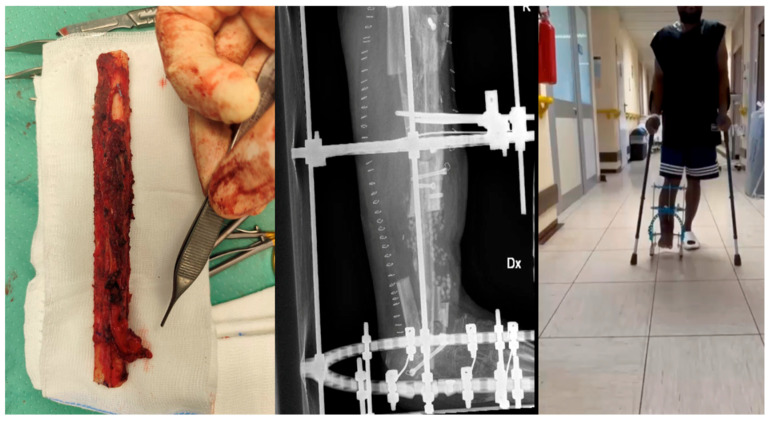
30-year-old male with chronic osteomyelitis of the mid-distal tibia following an undiagnosed and untreated closed trauma for nearly two years. Management included a staged orthoplastic approach: (i) first stage—tibial resection (15 cm) with talar resection, Masquelet technique, and external fixation; (ii) second stage—removal of cement and external fixator with initiation of bone transport; (iii) third stage—fistulectomy, debridement, and reconstruction with a free vascularized fibular flap combined with circular external fixation. Radiographic and clinical signs of healing were achieved, with satisfactory pain control.
